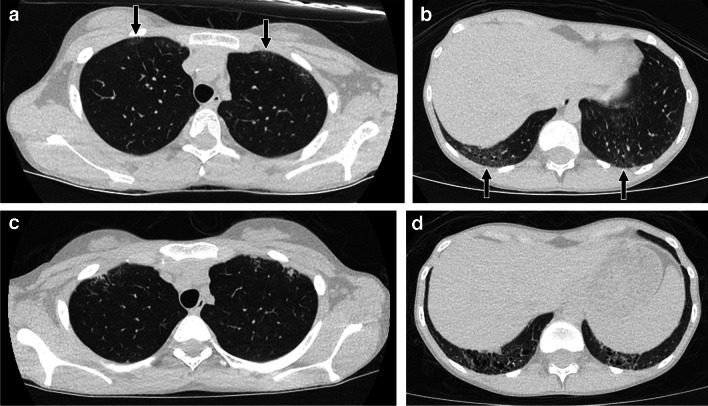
13-year-old female with systemic sclerosis. a, b Axial CT images without contrast showing subpleural ground-glass opacity, mild reticulation, and mild traction bronchiolectasis in the lower lobes and anterior upper lobes (black arrows) suggesting nonspecific interstitial pneumonia. c, d Follow-up axial CT images without contrast at 19 years of age showing increased reticulation and traction bronchiolectasis suggesting worsening fibrosis
